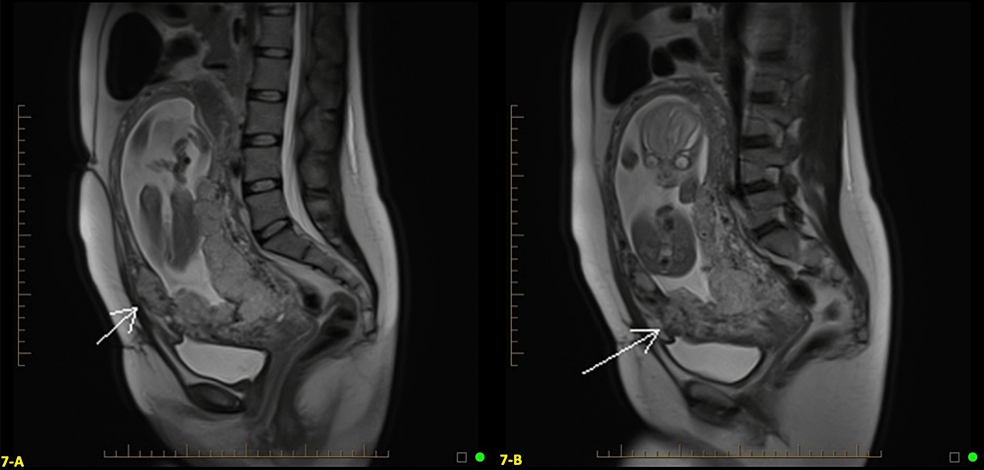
Sagittal half Fourier acquisition single-shot turbo spin echo (HASTE) Magnetic resonance imaging (MRI) findings at 23 weeks and one day gestational age (GA) A and B: Magnetic resonance imaging (MRI) at 23 weeks and one day gestational age (GA) confirming placenta previa with findings highly suspicious for placenta percreta. The placenta appears to involve the anterior abdominal wall in the midline, indicated by the white arrow (Image A), and involves the bladder (Image B).
